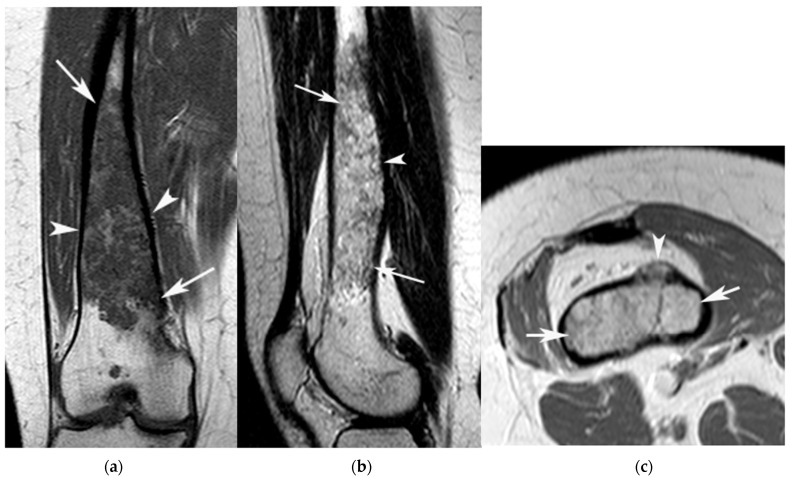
A 46-year-old female with Ollier disease and an ACT in the right distal femur. (a) Coronal T1W TSE, (b) sagittal T2W FSE and (c) axial PDW FSE MR images show an extensive chondral tumour (arrows) centred on the distal metametaphysis of the femur. The lesion fills the medullary cavity and results in bone expansion (arrowheads (a,b)). A small extra-osseous mass is also present (arrowhead (c)).
